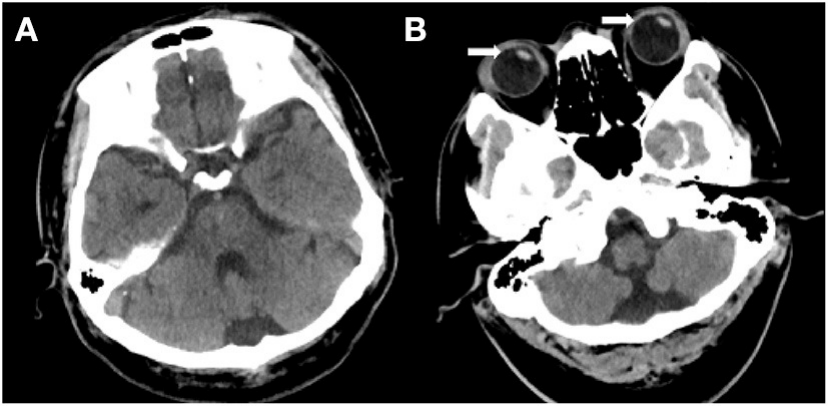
(A, B) Cranial computed tomography (CT) demonstrates no abnormality in the brain tissue. (B) A leftward conjugate ocular deviation is observed while the eyes are closed (white arrow).
